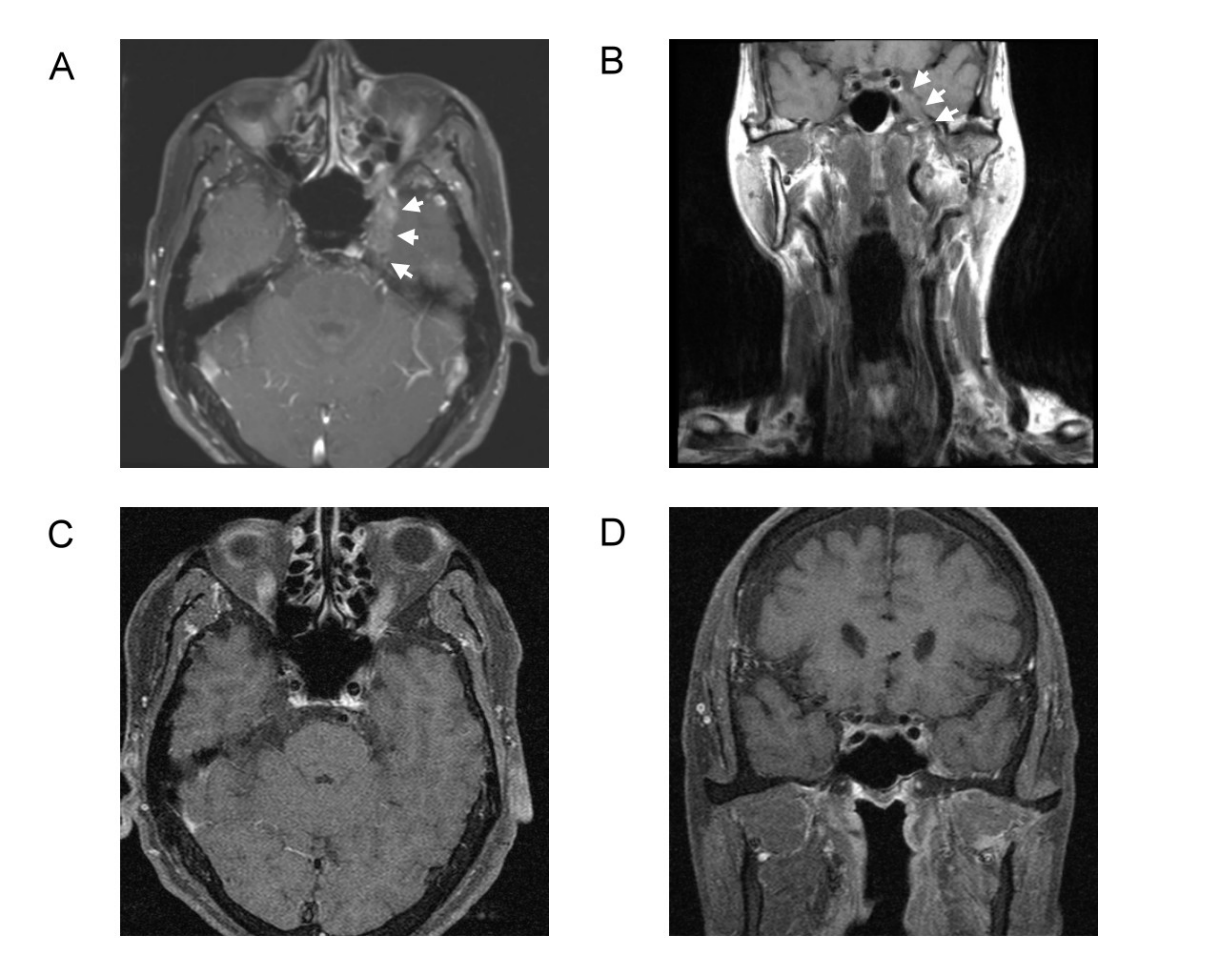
T1-weighted magnetic resonance imaging scans (A) Axial section shows irregular soft tissue mass in left cavernous sinus (arrows) before treatment, (B) Coronal section shows swelling of left parapharynx and masticator space and tumor invading into left cavernous sinus (arrows) before treatment, (C) Axial section shows remission of tumor 3 months after CCRT, (D) Coronal section shows remission of tumor 3 months after CCRT.
